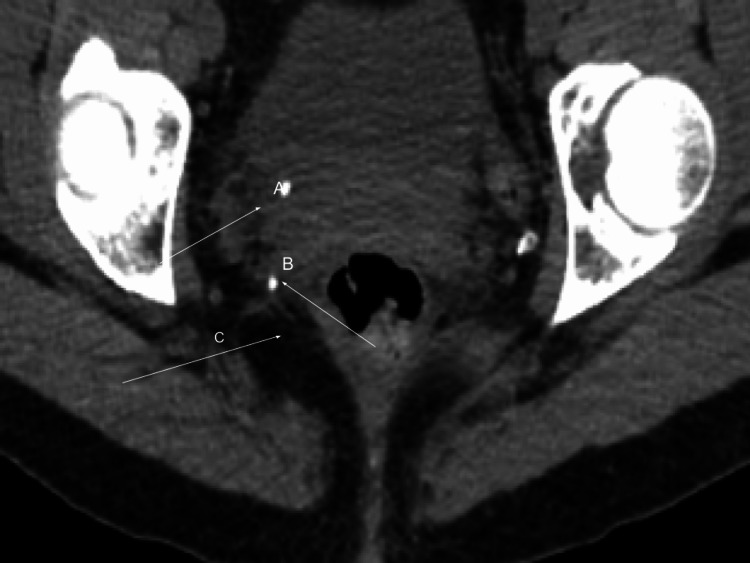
CT abdomen and pelvis without contrast The image shows 4-mm calcification in the region of the right ureterovesical Junction with right hydroureteronephrosis suspicious for obstructing renal calculus A, B: renal calculi; C: hydroureteronephrosis CT: computed tomography
